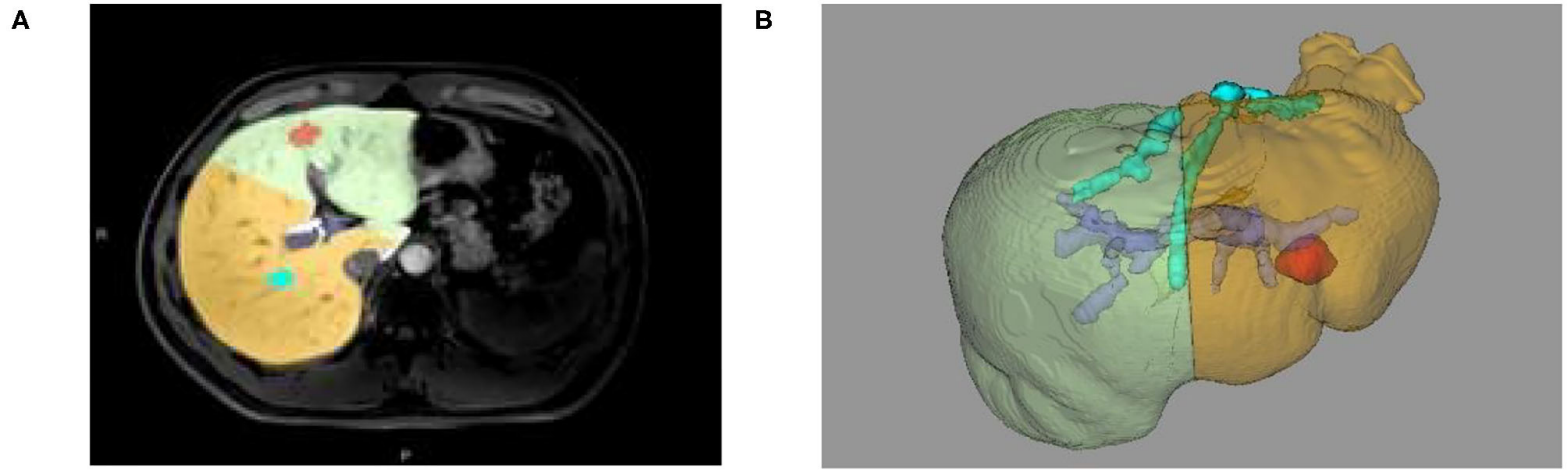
(A) Two-dimensional MRI enhanced image, (B) 3D reconstruction based on liver segmentation and tumor growth model on MRI data.
